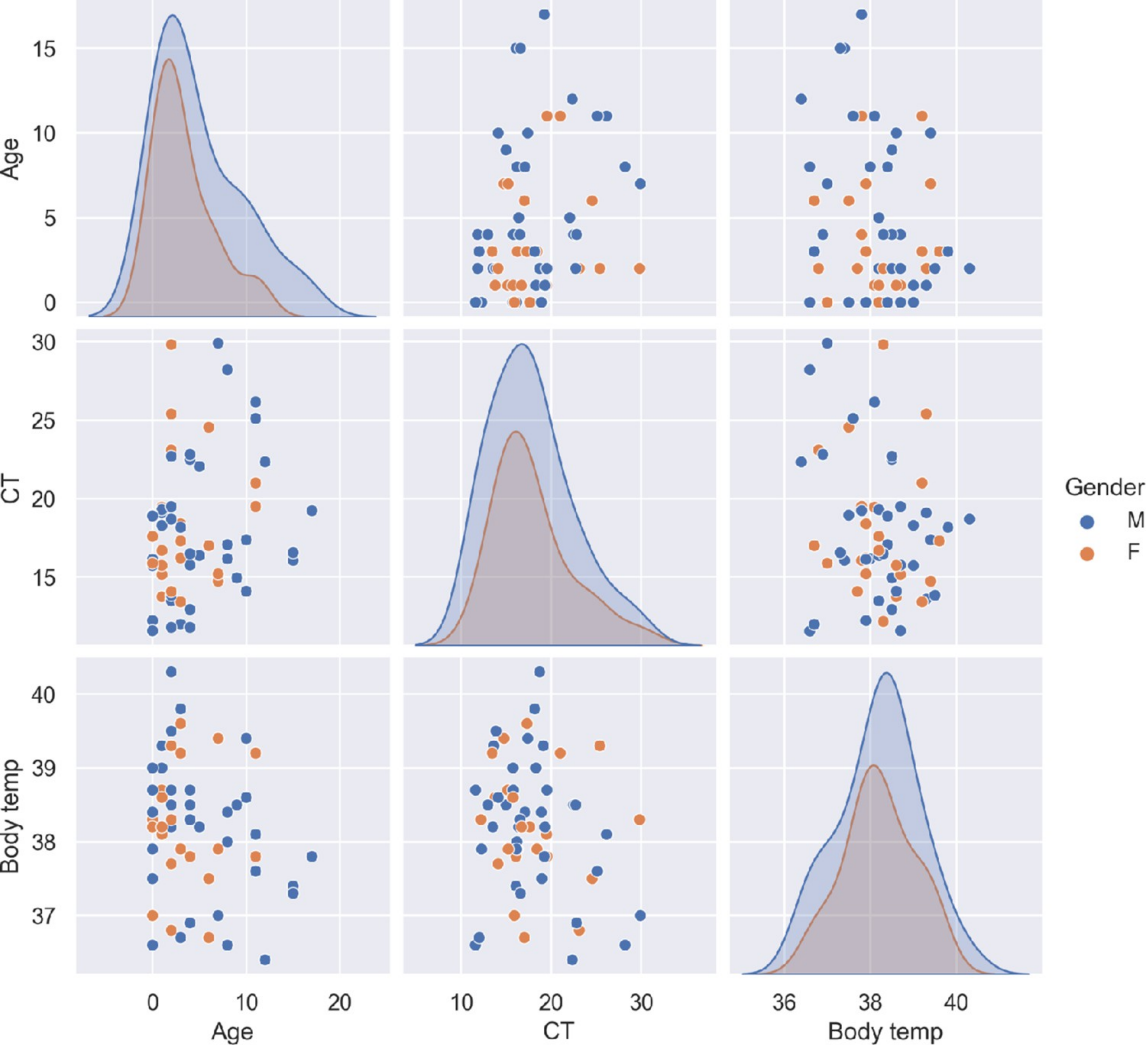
Distribution of CT values, body temperature and age of pediatric patients infected by Omicron variants BA.2.10.1 and BA.2.2. No sexual dimorphism was observed in this study.
